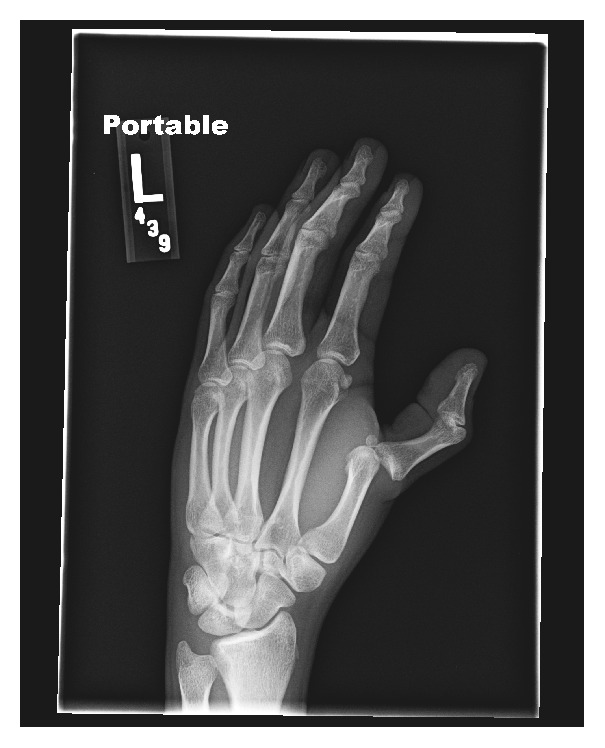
Radiograph demonstrating dislocated metacarpal-phalangeal joint of the first digit.
